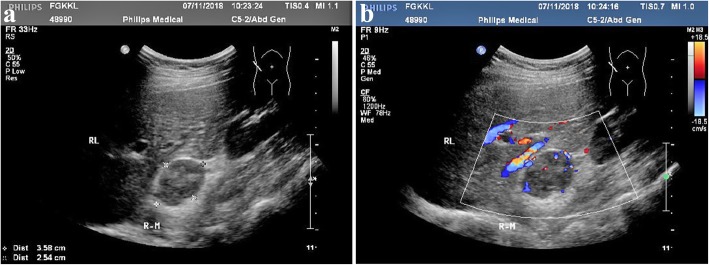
Ultrasound image showing a hypoechoic mass with a clear boundary in the right adrenal gland. The internal echo is heterogeneous (a). Color Doppler flow image showing a rich blood flow signal inside this mass (b)
